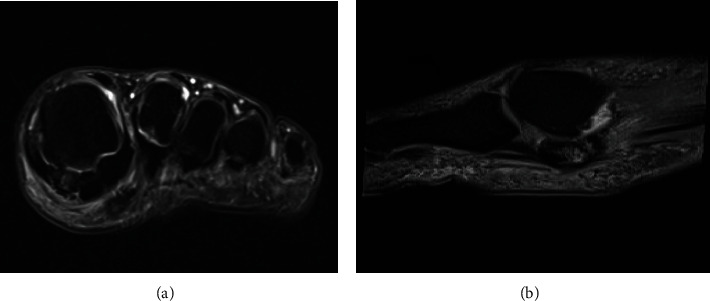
Magnetic resonance imaging of the left foot demonstrating bipartite medial sesamoid, diffuse homogeneous bone marrow edema of the medial sesamoid, periarticular soft tissue edema, and small glenosesamoid joint effusion ((a) coronal T2 with fat saturation, (b) sagittal T2 with fat saturation).
